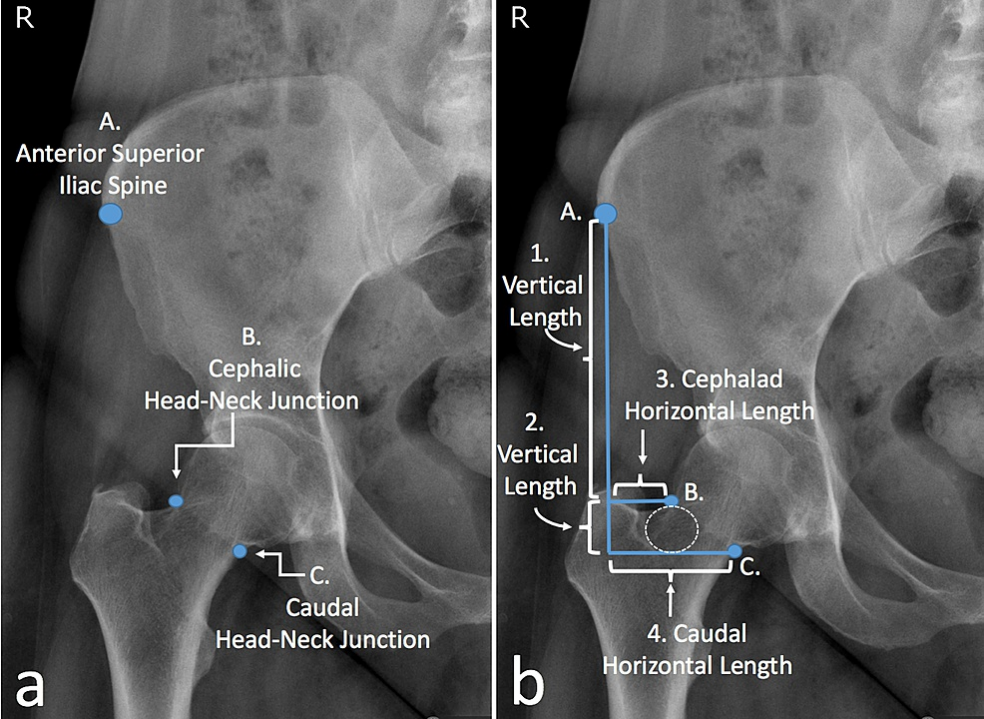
Identification of the anatomical reference points. On an anteroposterior hip radiograph with 100% magnification, three anatomical reference points are recognized: point A, the anterior superior iliac spine (ASIS), point B, the cephalic and point C, the caudal femoral head-neck junction (A). Three lines are drawn: a vertical line from the ASIS to the greater trochanter and two horizontal lines from points B and C connecting them to the vertical line. The preferred injection site is the anterolateral head-neck junction (circle, B).
